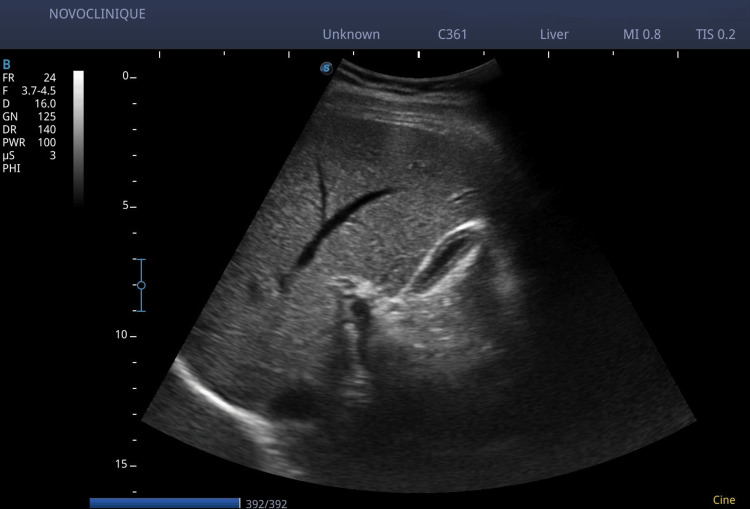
Biliary ducts ultrasound at three-month follow-up: complete resolution of the gallstones The postprandial gallbladder is visualized and emptied of bile, with no gallstones detected. There are no signs of pathological changes observed in the gallbladder or bile ducts.
